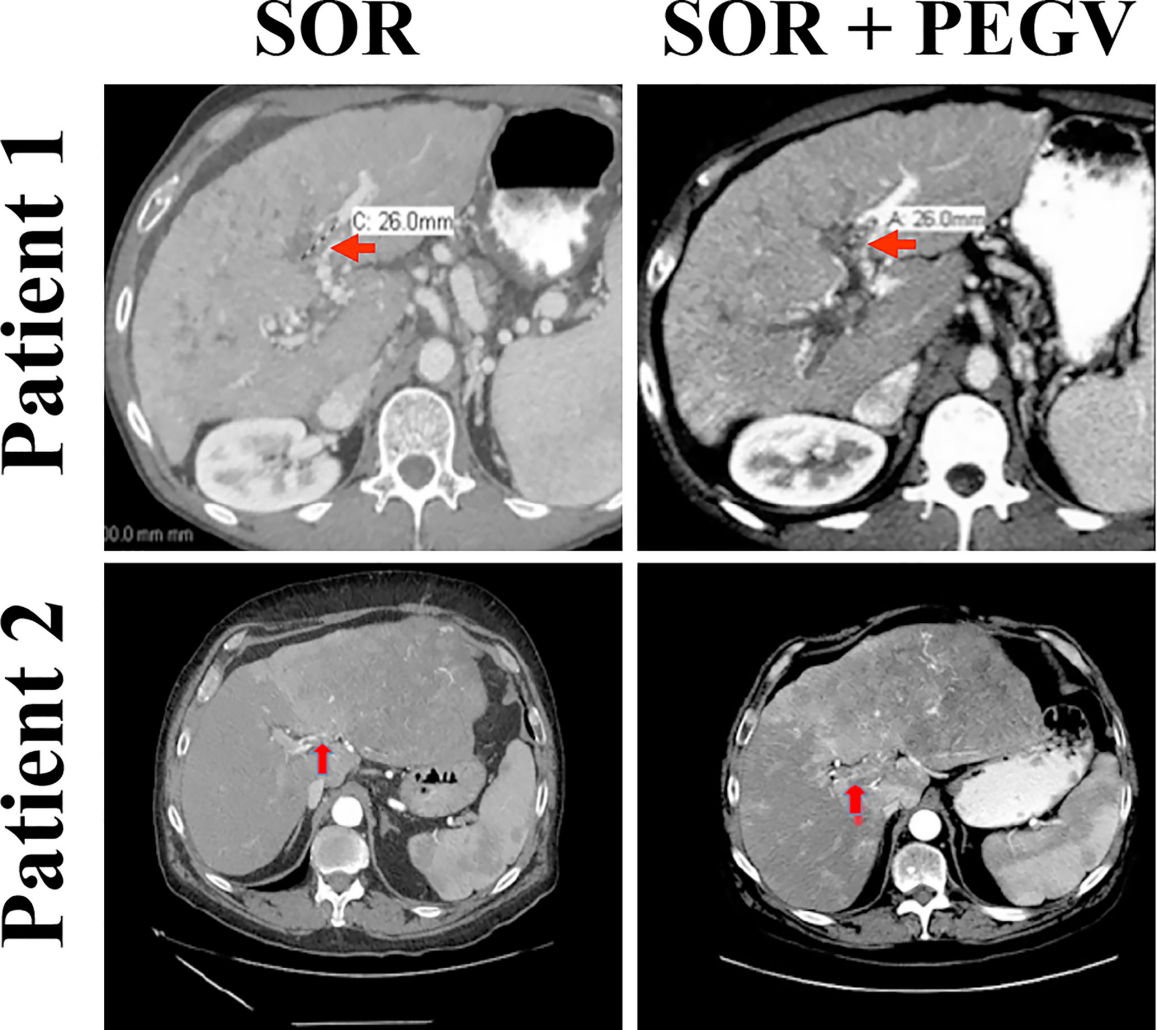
Blockade of GH/GHR signaling by pegvisomant causes tumor stability in HCC patients who develop sorafenib resistance. Two HCC male patients with clinical resistance and progression on sorafenib as well as with serum GH levels (5.3 and 3.7 µg/L) above those mandated by the FDA to allow administration of pegvisomant (normal level in males is ≤0.97 µg/L) were given pegvisomant, in addition to sorafenib, at a dose of 10 mg/kg/day subcutaneously. MRI scans after the addition of pegvisomant show disease stability. Patient 1 had a biomarker response in the form of a 40% decrease in AFP after combining pegvisomant with sorafenib. Patient 2 had low AFP (<20 µg/L) at baseline, which remained low on dual therapy.
